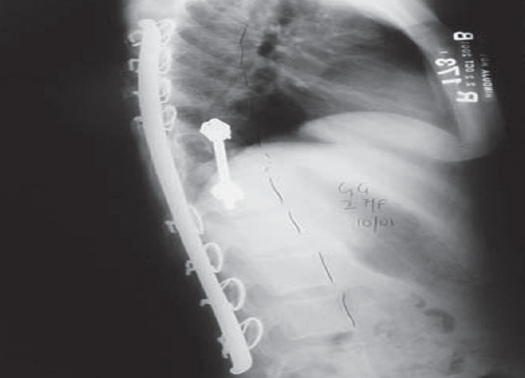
Postoperative X-ray in the same patient after combined posterior and anterior resection and reconstruction
